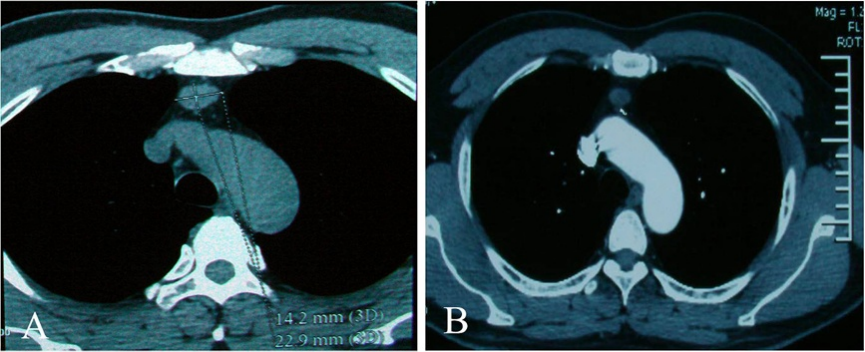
Computed tomography showed a tissue mass in the anterior mediastinum. (A) A plain chest scan showed a circumscribed soft tissue mass measuring 2.2 × 1.4 cm in size. (B) A contrast-enhanced scan showed a demarcated tissue mass with no calcification.
